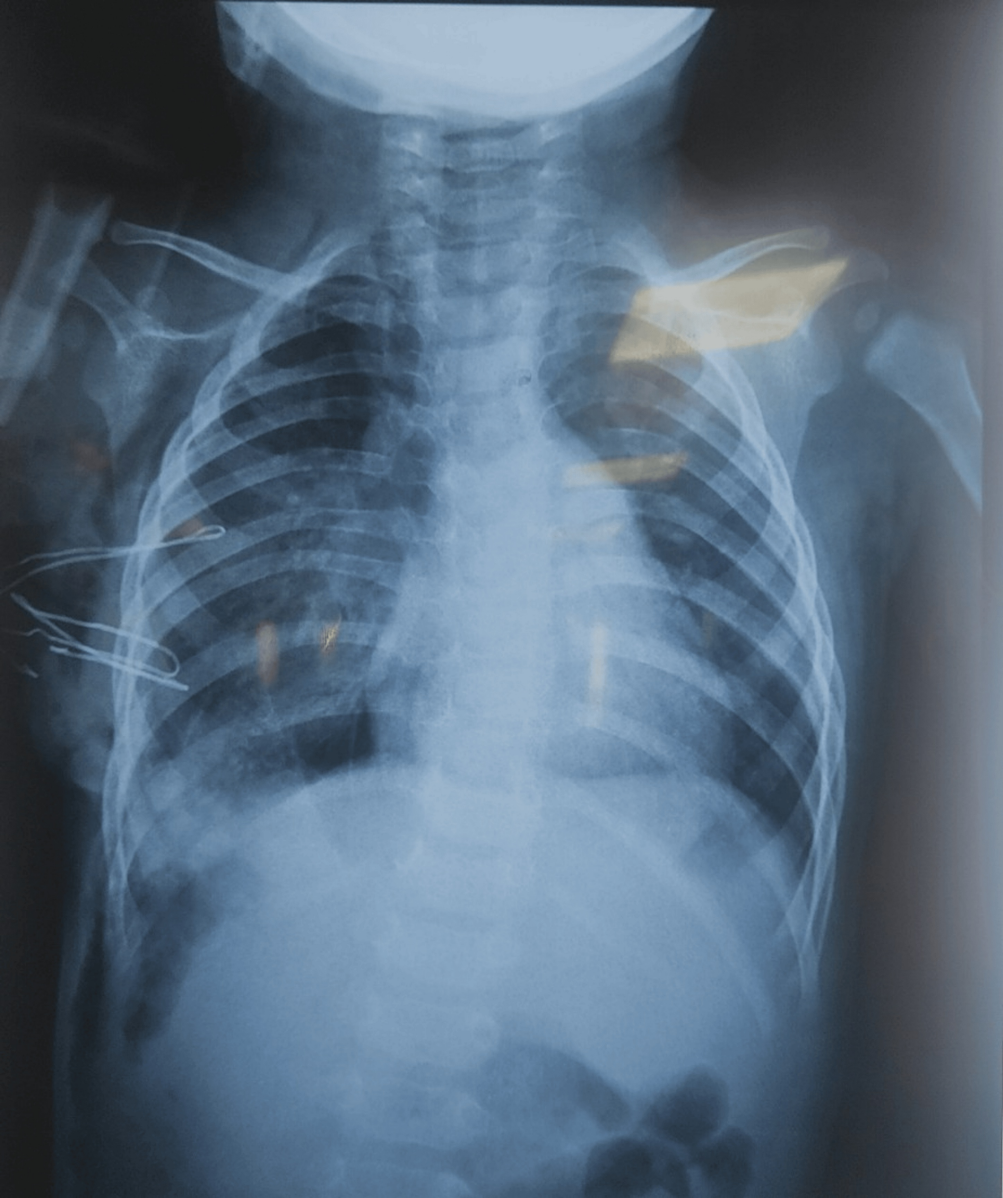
Postoperative chest X-ray Frontal chest radiograph showing corrected right hemidiaphragm position after plication with partial lung re-expansion and a mild right pneumothorax. The chest tube is visible in situ.
